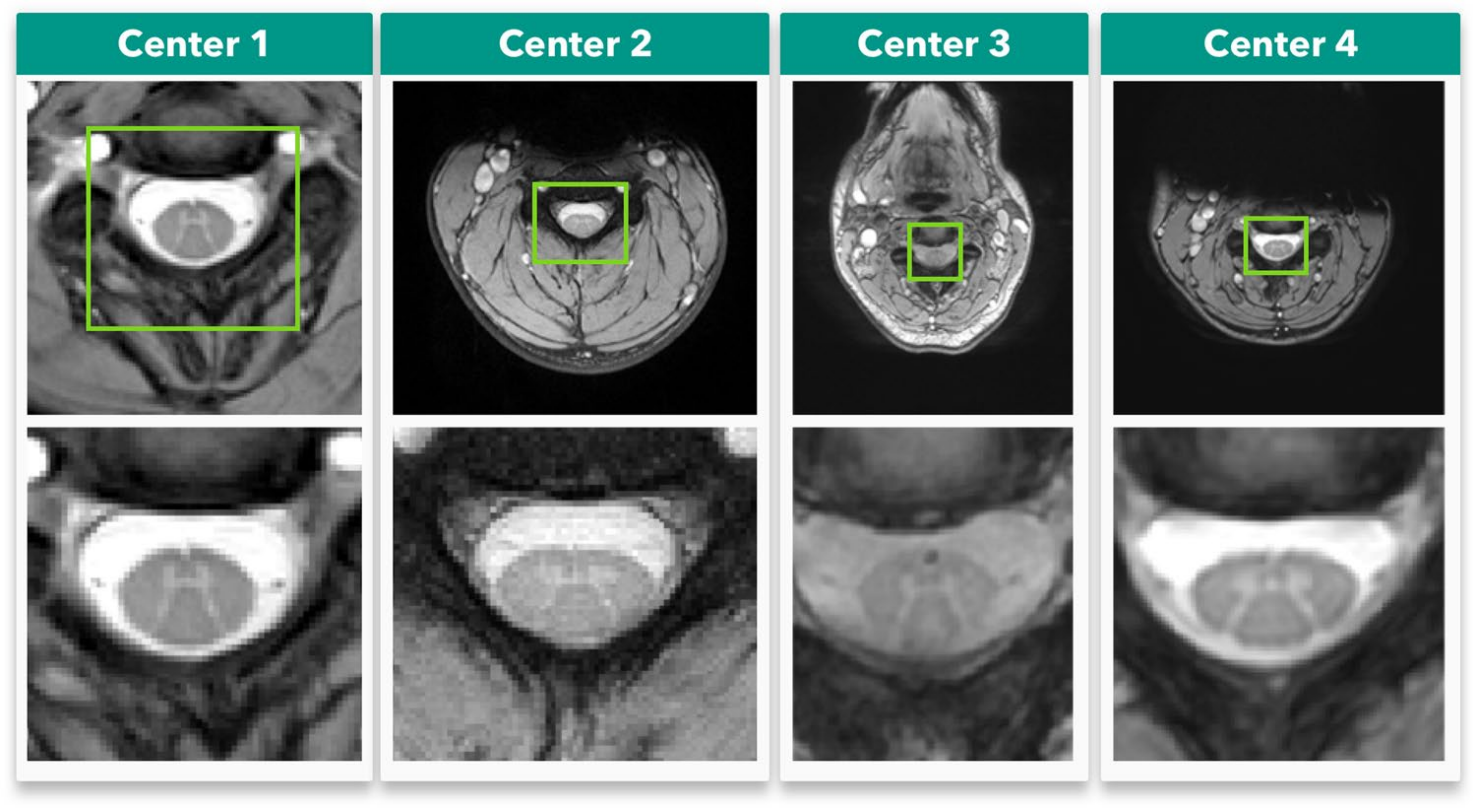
In vivo* axial-slice samples from four centers (UCL, Montreal, Zurich, Vanderbilt) that collaborated to the SCGM Segmentation Challenge^6^. Top row: original MRI images. Bottom row: a crop of the spinal cord (green rectangle).
